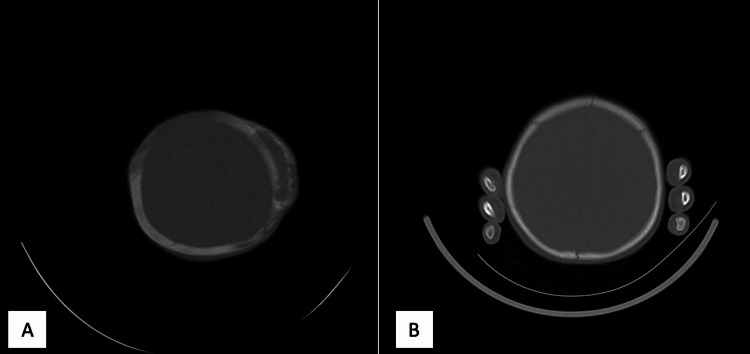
(A) An ossified cephalohematoma was seen in the left parietal region in a two-month-old baby. (B) Ossification was completely resorbed in the control CT taken at the ninth month.
